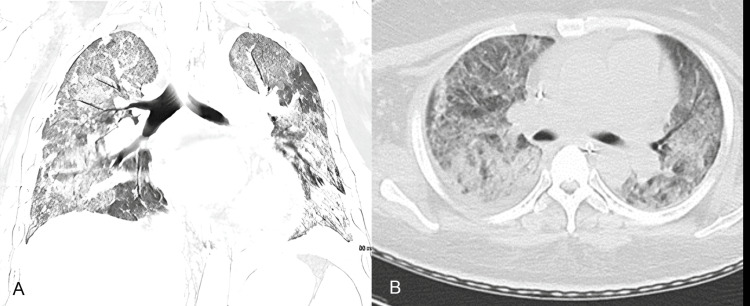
Computed tomography images in the axial plane (A) and coronal plane (B) of the chest, demonstrating bilateral ground glass opacities throughout both lungs. These images represent lung windows on CT scan.
